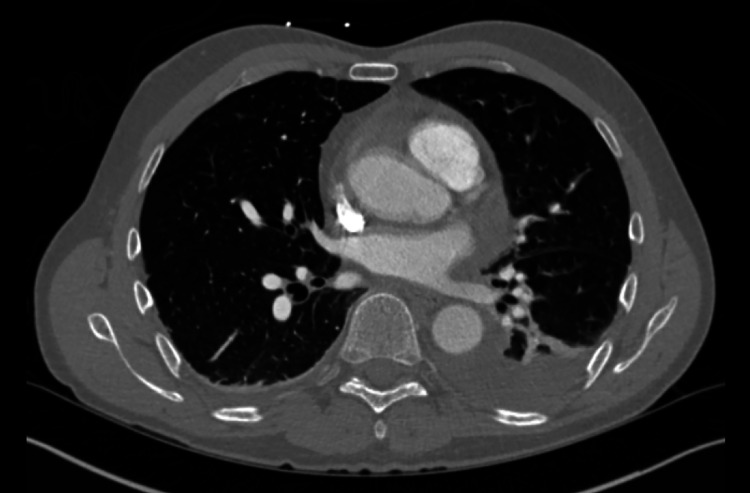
Computed tomography with small pericardial effusion, pericardial thickening, and left pleural effusion
